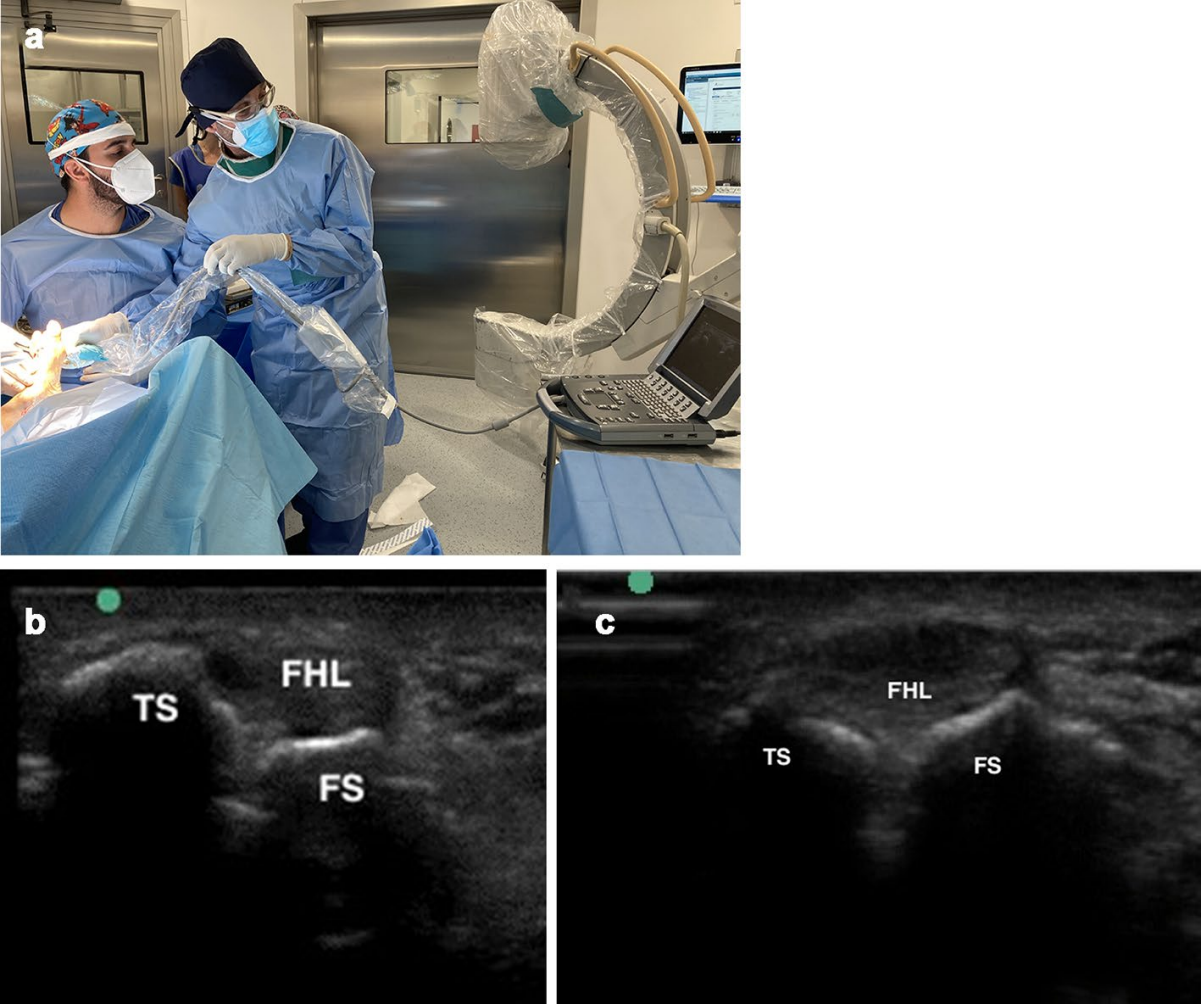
a An ultrasound view is carried out for checking the final position of the sesamoids and its level respect to lesser metatarsal heads. b Sonographic view of the sesamoid luxation in the context of hallux valgus. Note the tibial sesamoid (TS) and the fibular sesamoid (FS) positioned at different levels in axial plane and the FHL displaced laterally. c Sonographic view of both sesamoids bones and the flexor hallucis longus (FHL) between tibial sesamoid (TS) and fibular sesamoid (FS)
